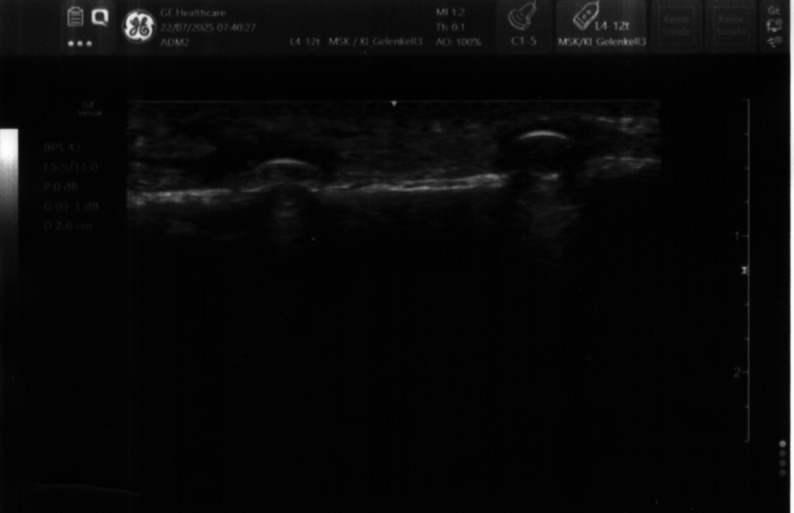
The image shows the longitudinal sonographic view of two syndesmotic screws in situ, appearing as hyperechoic structures with comet-tail artifacts. The image corresponds to a 49-year-old female patient (BMI 26.1) with a history of ankle sprain and syndesmotic injury treated by syndesmotic screw fixation
